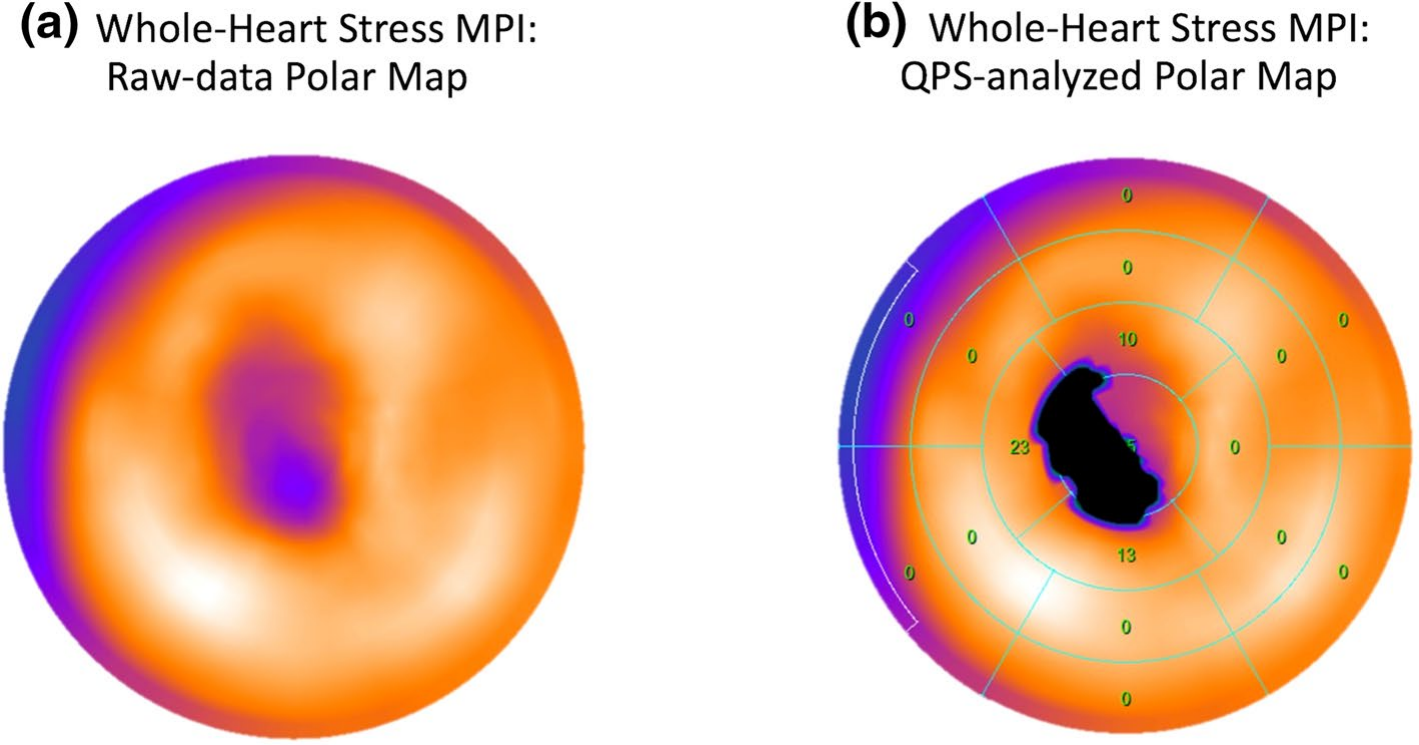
Example stress SPECT MPI data for a case wherein ischemia is detected on the basis of the whole-heart TPD score but 3-slice TPD measurement fails to detect the severe ischemia. a Polar map with raw perfusion data; b automatically analyzed whole-heart polar maps generated in the QPS software (whole-heart stress TPD = 6.0%). The presented case is the stress MPI data for a 58-year-old female patient showing a severe apical perfusion defect, which is consistent with her invasive angiography results that indicated 90% LAD stenosis. In b, the measured perfusion defect region is shown in black and the numbers in each segment indicate the corresponding defect extent (in percentage) for that segment. The 3-slice stress TPD (computed from the conventional short-axis slice positions as shown in Fig. 2) was 0.9%, which is below the 3% abnormality threshold. *LAD* left anterior descending, *MPI* myocardial perfusion imaging, *TPD* total perfusion deficit
